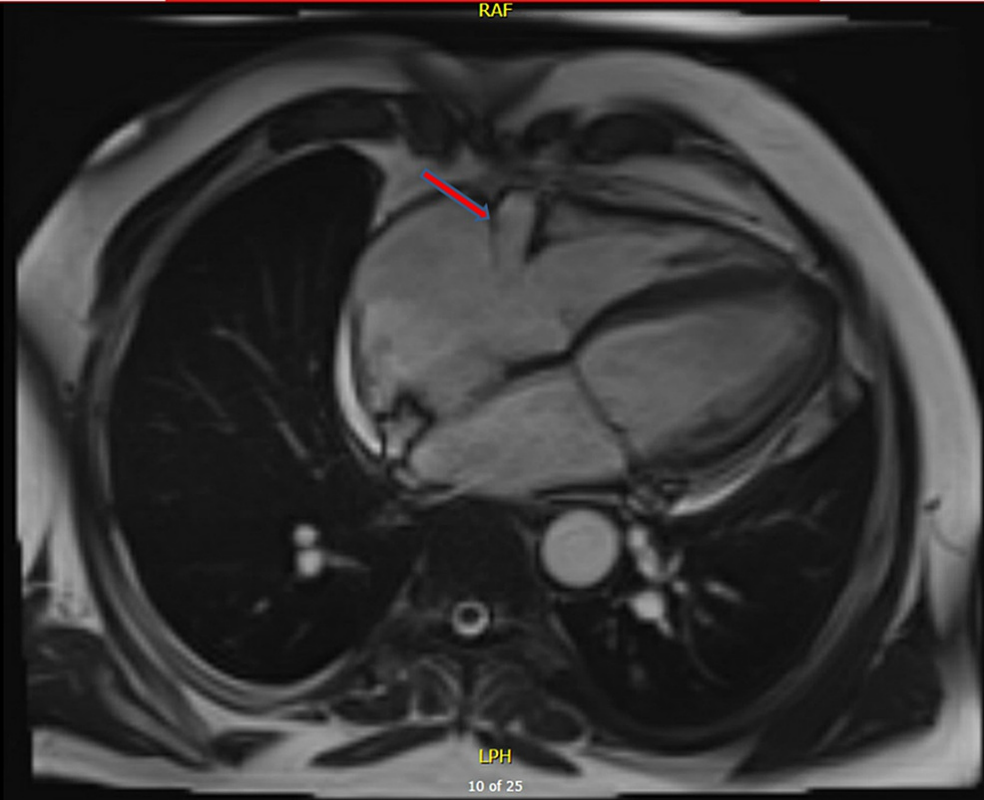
Cardiac MRI showing the fistula and shunting (red arrow).
